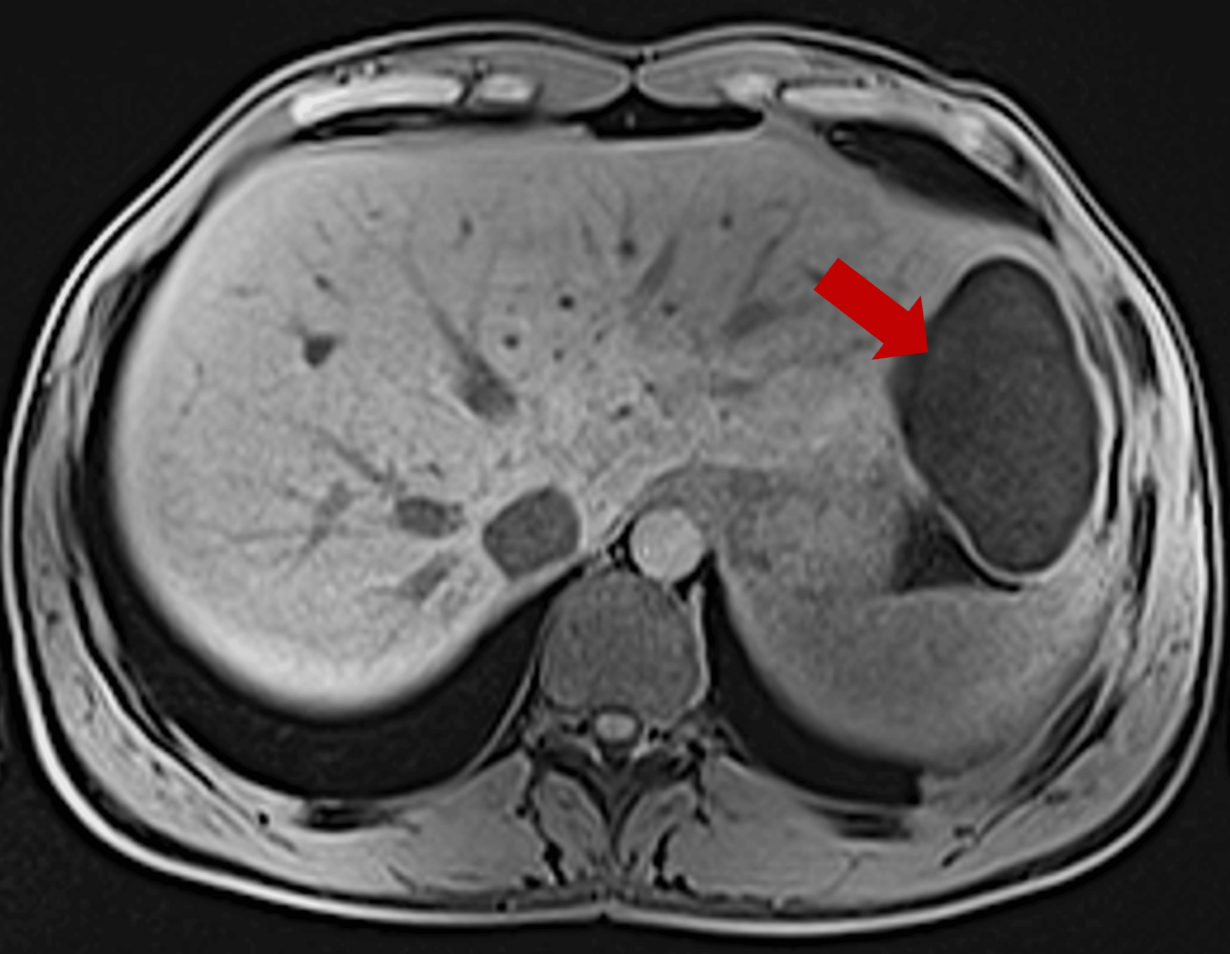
Abdominal MRI (axial view). The red arrow shows a hepatic hydatid cyst in the left lobe. MRI, magnetic resonance imaging
